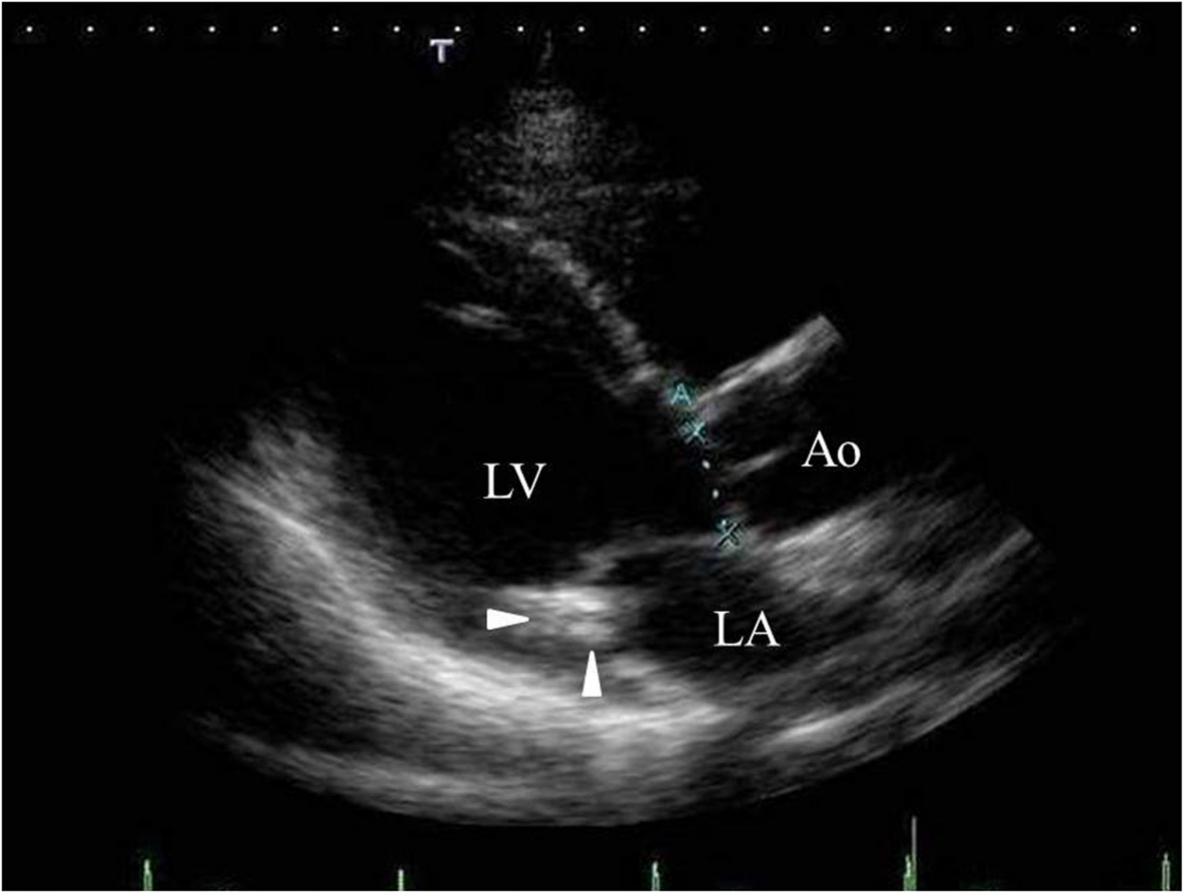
Two-dimensional transthoracic echocardiogram. Parasternal long-axis view demonstrating the high echoic mass (arrow heads) attached to the posterior mitral valve leaflet. LV, left ventricle; LA, left atrium; Ao, aorta.
